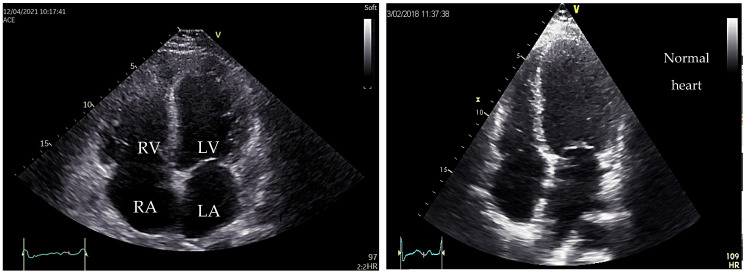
Two-dimensional echocardiography, apical four-chamber view showing biventricular dilation with spherical geometry and bilateral atrial enlargement, versus normal heart to the right side of the image.
